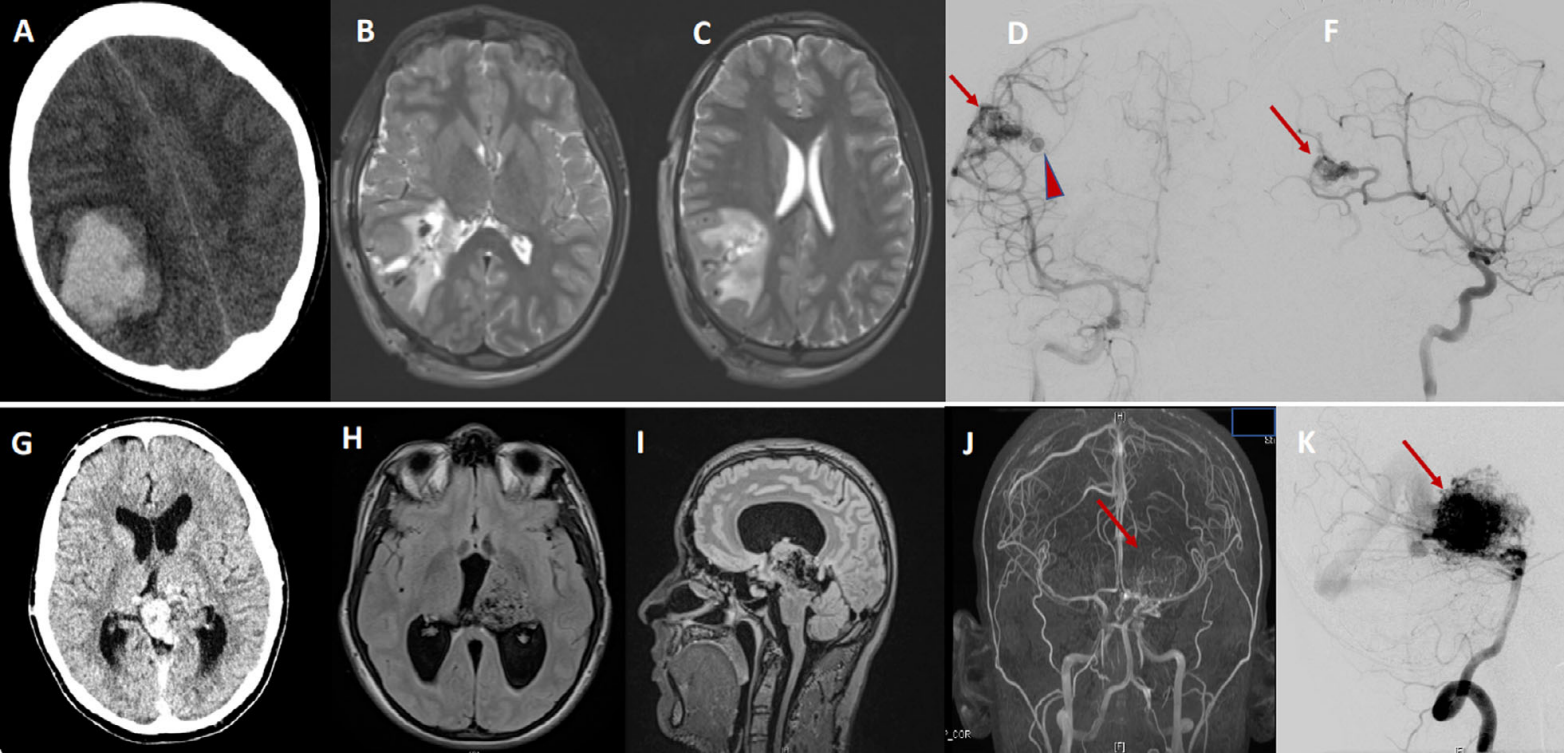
Top Row: A 12‐year‐old boy presenting with acute onset loss of consciousness, left hemiparesis, and left hemibody sensory loss. Axial noncontrast CT (A) showed acute right parietal intracranial hemorrhage with midline shift. Axial MRI T2W images postemergent evacuation of the hematoma, with resolution of mass effect (B, C). Right internal carotid artery angiogram demonstrating a small right parietal arteriovenous malformation (AVM) (arrow), with an intra‐nidal aneurysm (arrowhead) compatible with the rupture site (D, F). Bottom Row: An 18‐year‐old man with headaches, difficulty walking, and hearing a whooshing sound after taking a flight for the first time. Noncontrast CT (G) and brain MRI (H, I) showed a large left thalamic mass causing moderate obstructive hydrocephalus. MRA (J) showed a large vascular lesion with numerous enhancing tortuous vessels (arrow) in the left thalamus extending inferiorly to midbrain. Vertebral angiography (K) confirmed AVM with the dominant supply from the left posterior cerebral artery and deep venous drainage.
